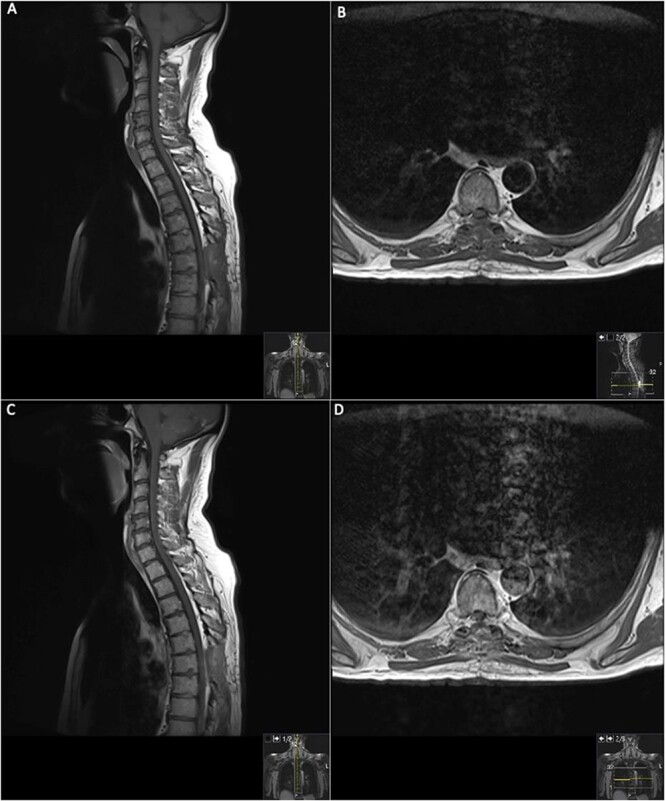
19 days post operative MRI; T1 pre-contrast sagittal (A) and T1 pre-contrast axial (B) show evidence of surgery at T6. Post-contrast T1 sagittal (C) and axial (D) show evidence of a small ‘fleck’ of dural enhancement, however, provide no evidence of any definite residual lesion.
